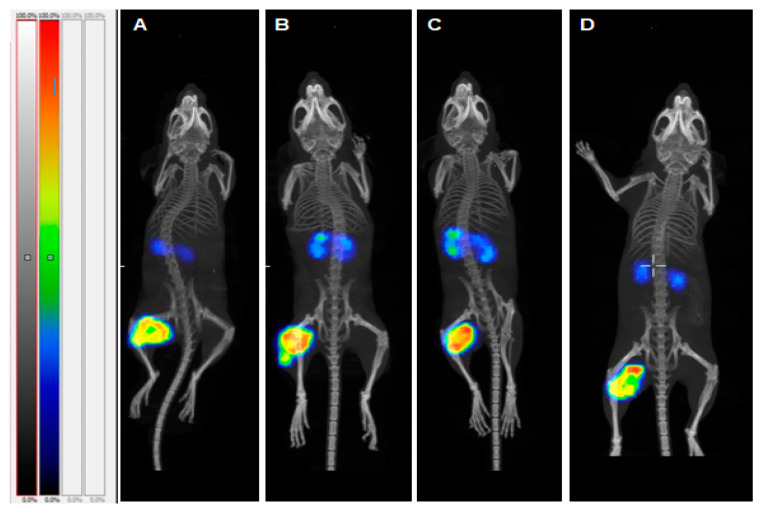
SPECT/CT imaging (maximum intensity projection) of HER2-expressing SKOV3 xenografts for pretargeting using (A) [^177^Lu]Lu-*HP16*, (B) [^177^Lu]Lu-*HP17*, (C) [^177^Lu]Lu-*HP18*, and (D) [^177^Lu]Lu-*HP2* 4 h after injection. The color scale bar represents a relative activity normalized to the activity in tissues with the highest uptake in each image.
